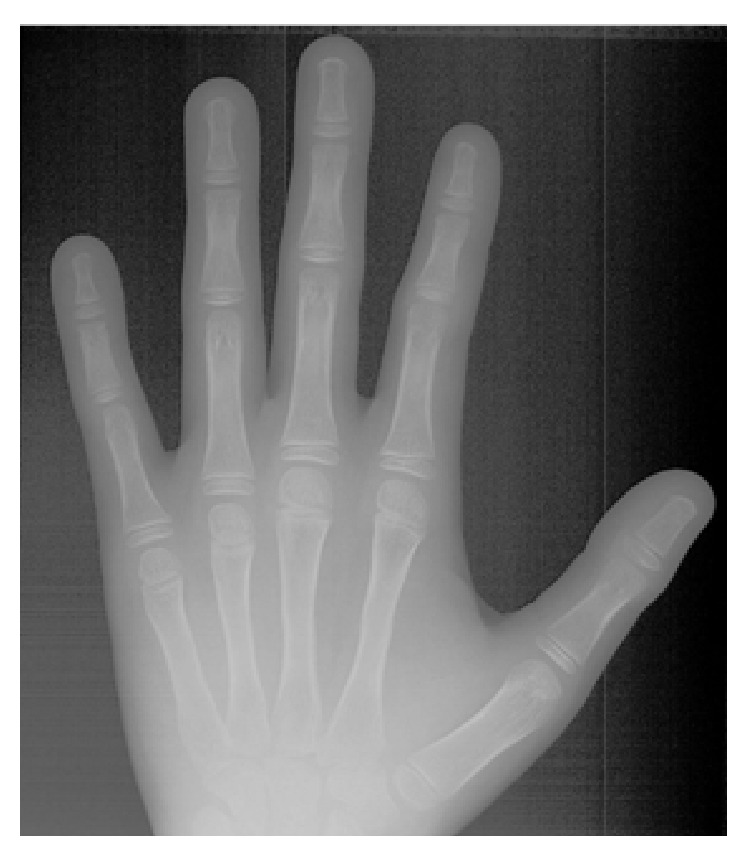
Initial hand-wrist radiograph.
